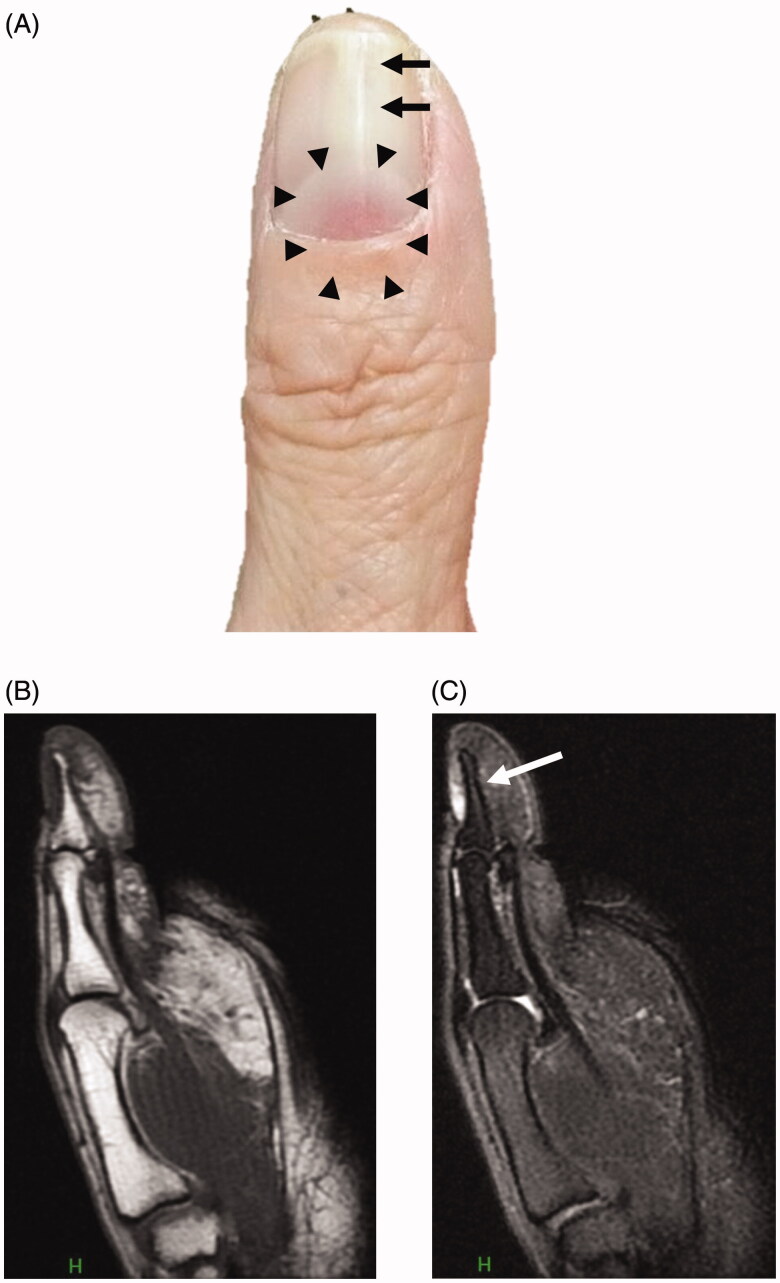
Clinical findings and MRI images. (A) A reddish mass of 4-mm diameter is seen under the nail plate (area surrounded by the triangle mark). Distal nail splitting is observed in the left thumb. (B) T1-weighed magnetic resonance image shows tumor with normal intensity. (C) T2-weighed magnetic resonance image shows high-intensity lesion. Flow void is indicated by an arrow. MRI: magnetic resonance imaging.
